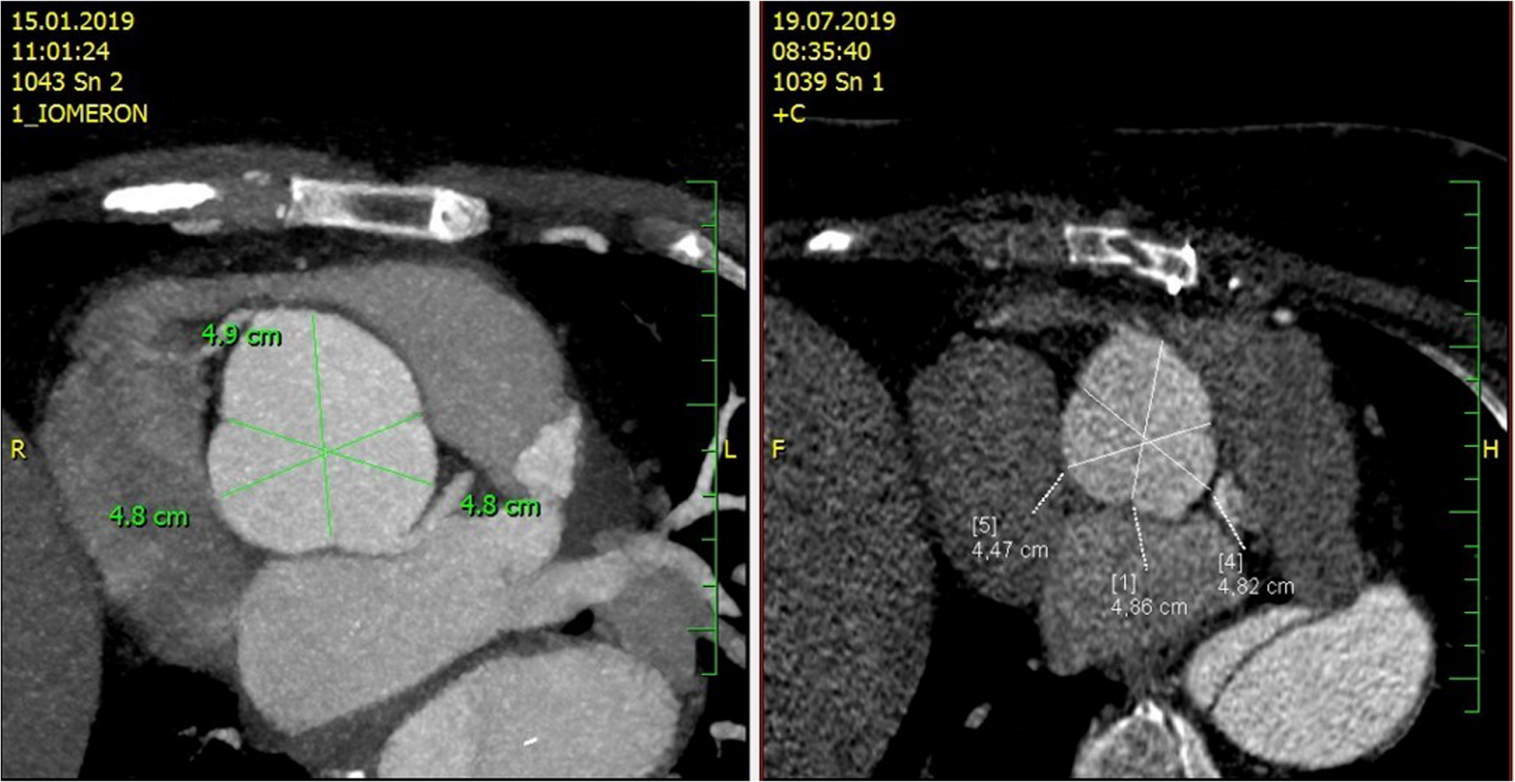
CT AG before PEARS and at 14 months after PEARS procedure
